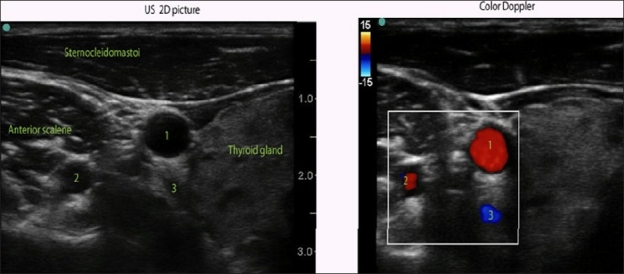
Color Doppler over 2-D US of the anterolateral neck area at C6/C7 level. While looking only at the 2-D US image, one may confuse vertebral artery (2) with a nerve root. Both may look the same on 2-D US. Color Doppler helps to distinguish nerve roots from blood vessels. 1) Carotid artery; 2) Vertebral artery; 3) Inferior thyroid vein
